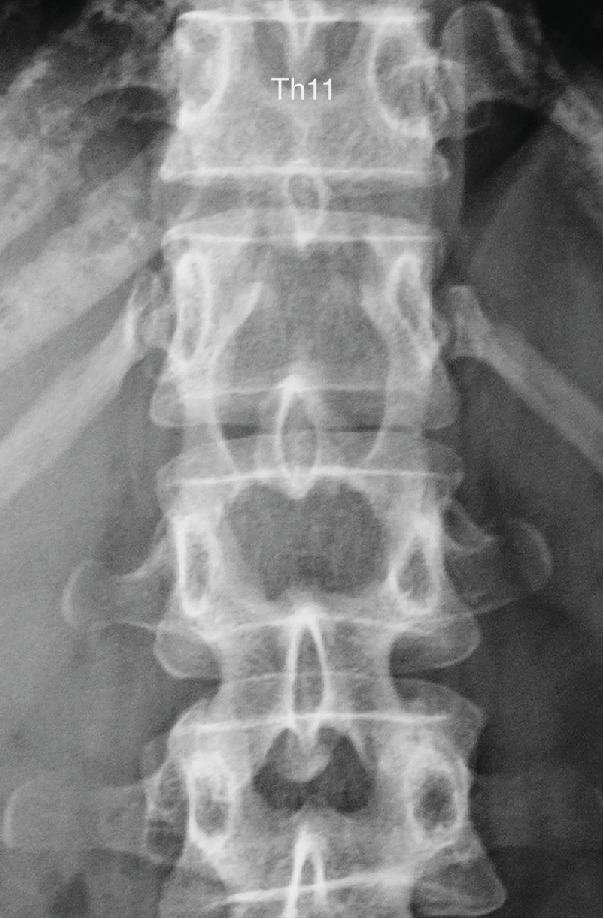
Plain radiography showed expansion of the space between the arches at T12/L1 and L1/2.
